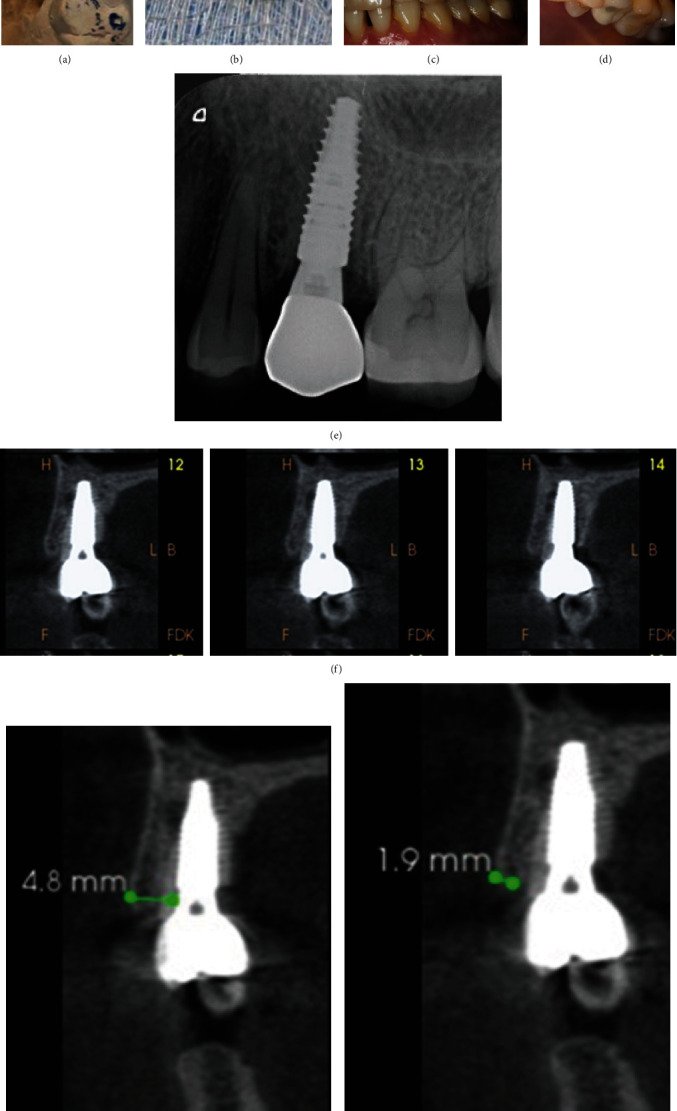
Zirconia crown cemented onto titanium abutment (a) and (b), lateral and occlusal views of crown screwed into the implant (c) and (d), postoperative PA (e), F–L radiographic sections (f), F–L section verifying width of hard facial tissues (g), and F–L section verifying width of facial plate crest (h).
